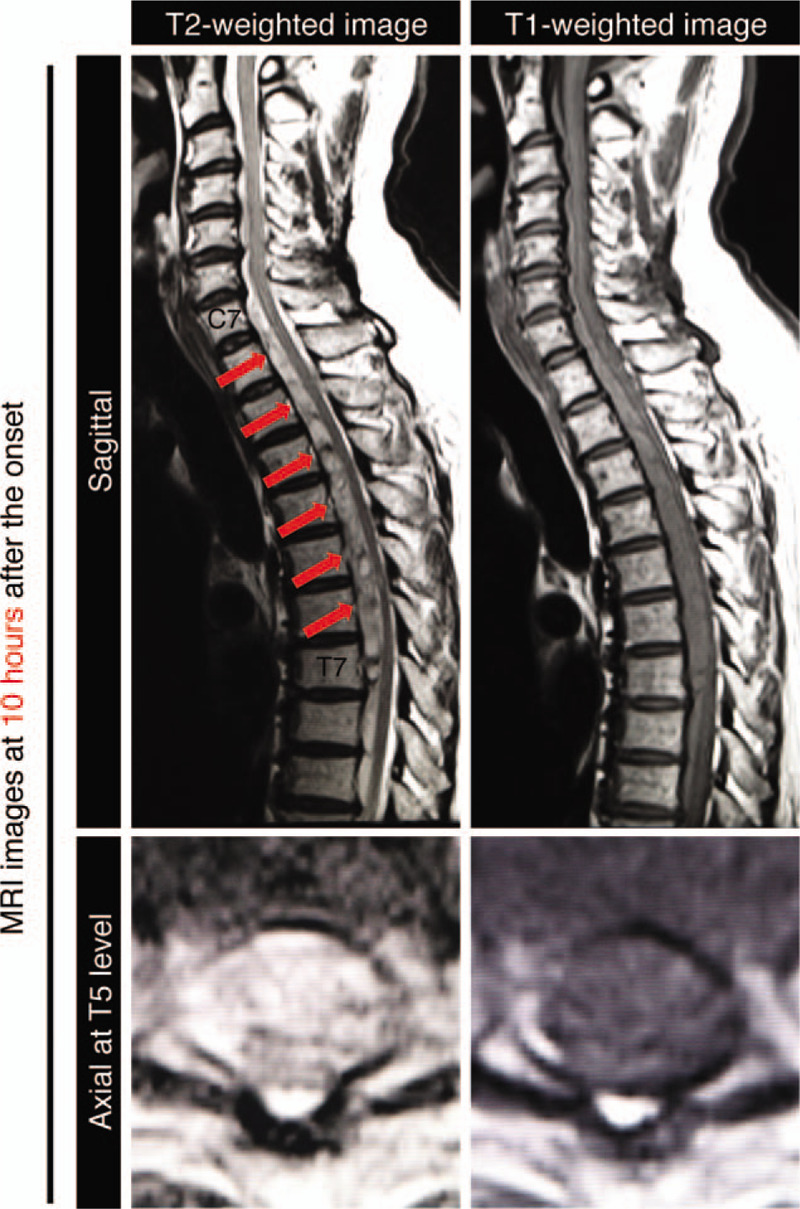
Magnetic resonance imagings (MRIs) of cervicothoracic spine at 10 hours after the onset of presenting paraplegia. T2-weighted and T1-weighted images revealed a long segment lesion of mixed isointensity and hyperintensity signals from C7 to T7. MRI images of the axial section at the T5 level showed the displacement of the spinal cord due to the lesion.
